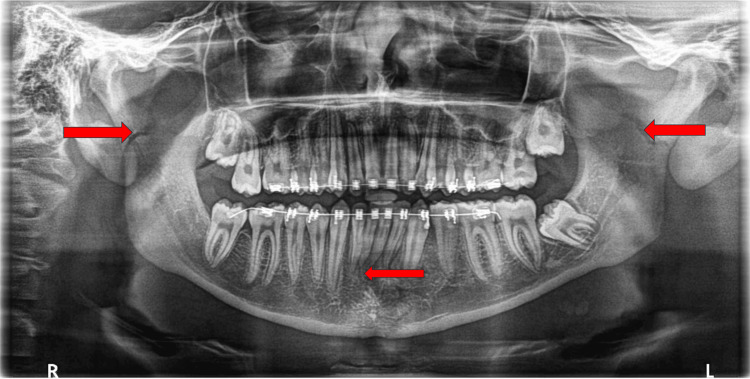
Orthopantomogram (OPG) showing a hairline fracture at the symphysis region of the mandible along with bilateral subcondylar fractures (red arrows).
